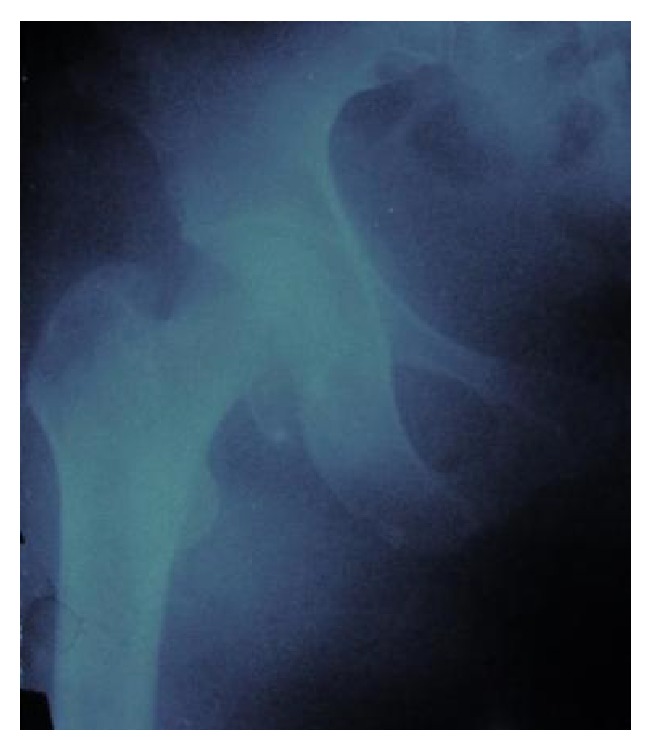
Radiograph revealed the fracture of the femoral head.
